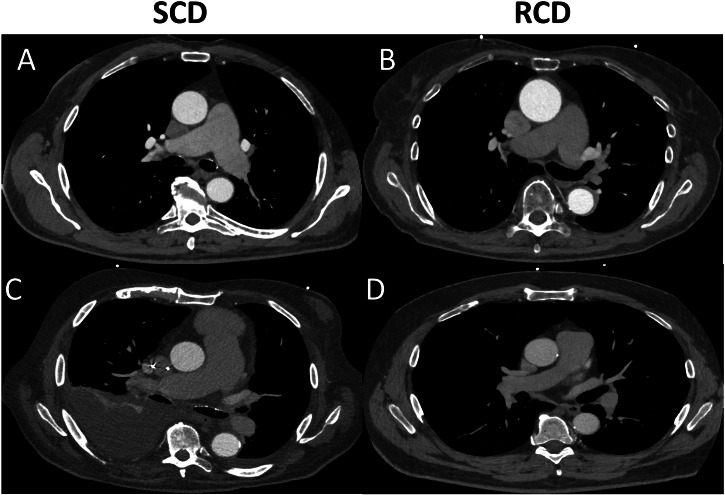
Representative polychromatic axial images (window width/window level: 700/80 HU) of a female (A) and a male patient (C) acquired with standard contrast media dose (SCD), and BMI-matched female (B) and male (D) patients with reduced contrast media dose (RCD), acquired with a photon-counting detector CT (PCDCT)
